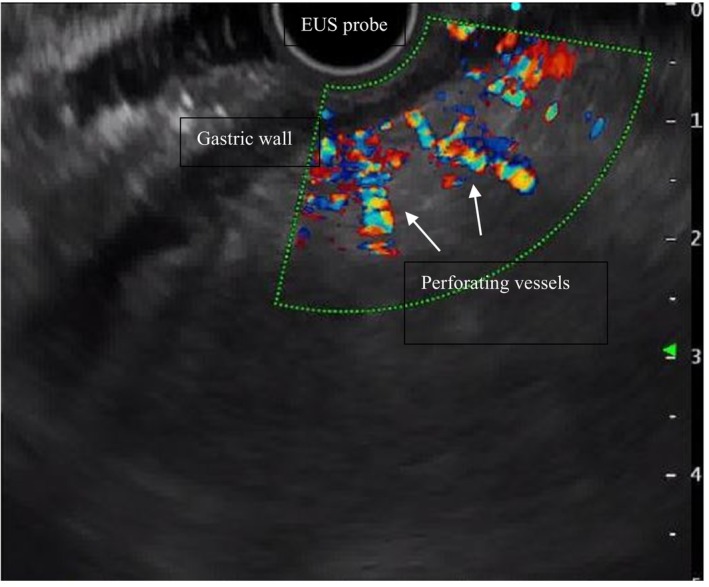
Evaluation of perforating vessels of the gastric wall on colour Doppler during endoscopic ultrasound in a patient with liver cirrhosis and portal hypertension.
